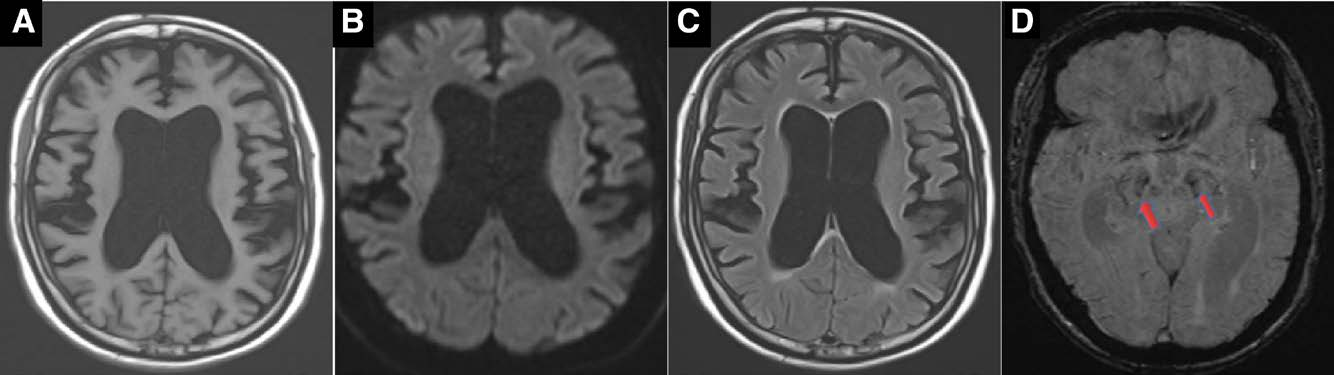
Brain MRI. Substantial ventricular enlargement and cerebral atrophy on T1-weighted images (A), diffusion-weighted images (B), and fluid-attenuated inversion recovery sequences (C), with susceptibility-weighted images displaying indistinct bilateral substantia nigra (D, arrows).
